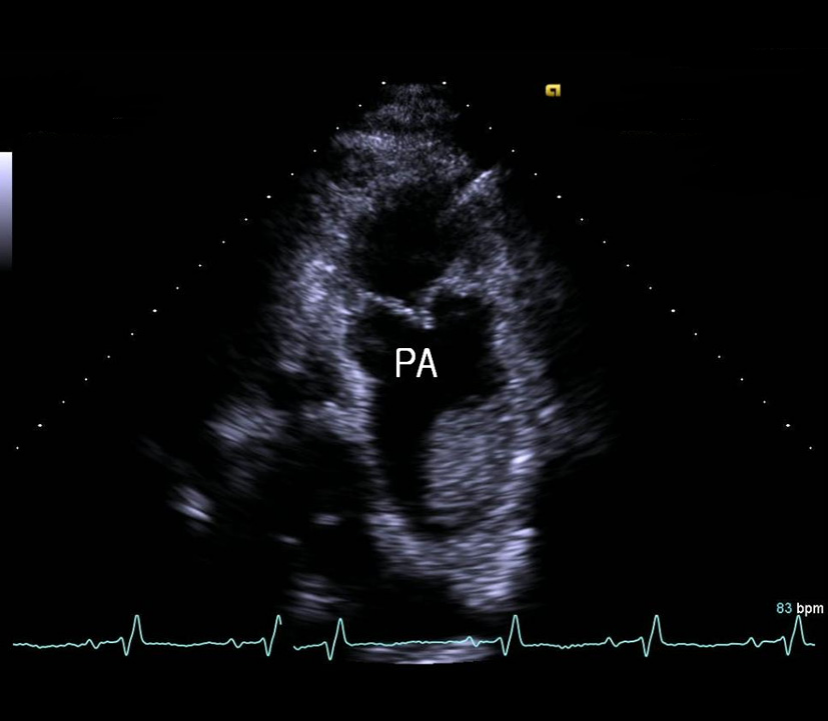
Transthoracic echocardiography at the modified parasternal short axis view showed proximal pulmonary artery thrombus. PA, pulmonary artery.
